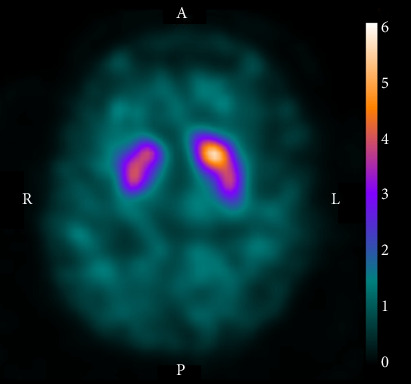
Dopamine transporter-single photon emission computed tomography findings. The tracer accumulation was decreased in the right striatum. The scale bar indicates the extent of tracer accumulation when the mean background concentration is postulated as 1.
